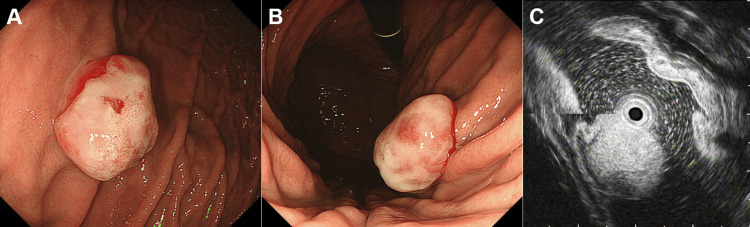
Endoscopic findings of the gastric lesion. Esophagogastroduodenoscopy reveals a reddish, semi-pedunculated elevated lesion on the anterior wall of the upper gastric body, covered with a whitish coating (A, B). Endoscopic ultrasonography demonstrates a hyperechoic mass extending from the mucosal to the submucosal layer (C).
